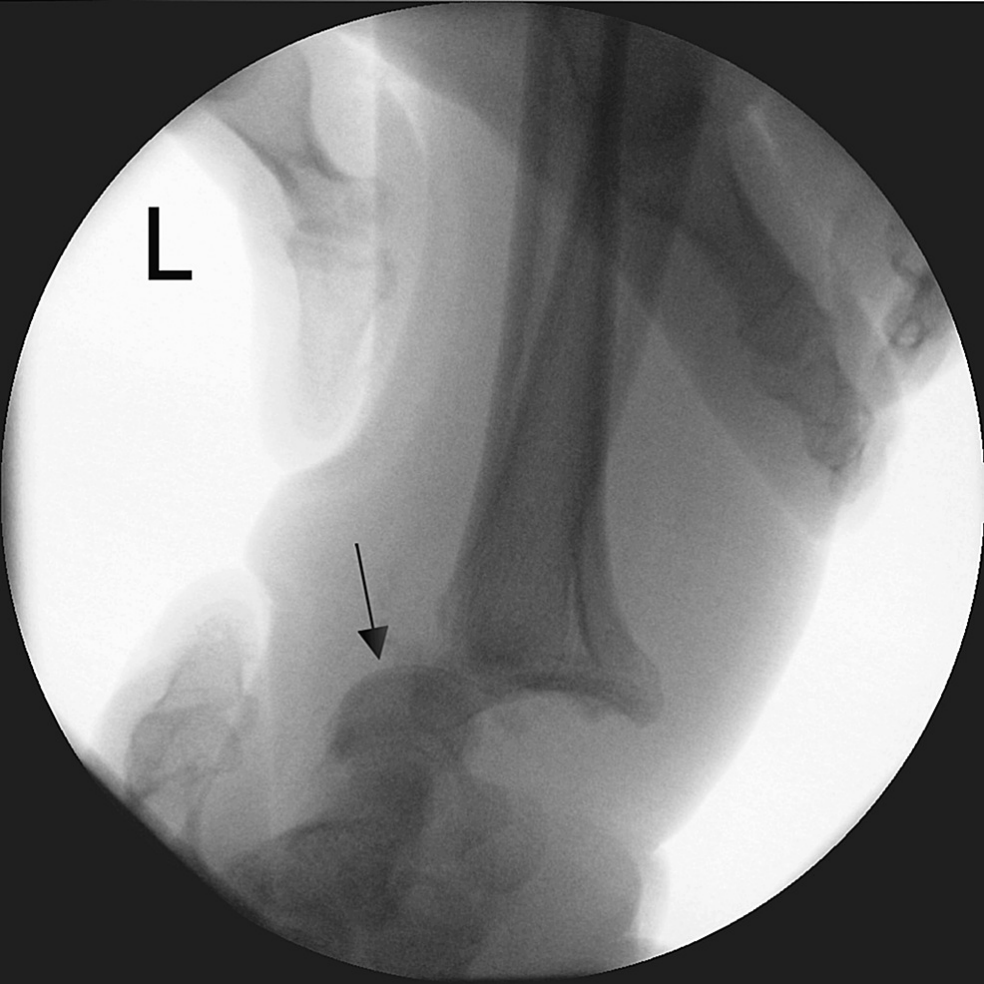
Perioperative X-rays of the wrist The lateral view shows visible radiocarpal dislocation.
